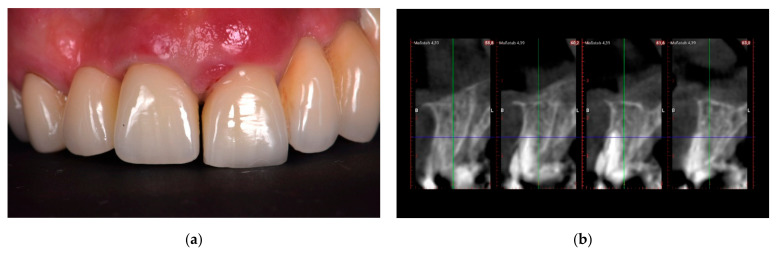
First clinical case. Pre-operative intra-oral view of a patient: (a) frontal view of the compromised tooth #21; (b) pre-operative CBCT radiographs.
